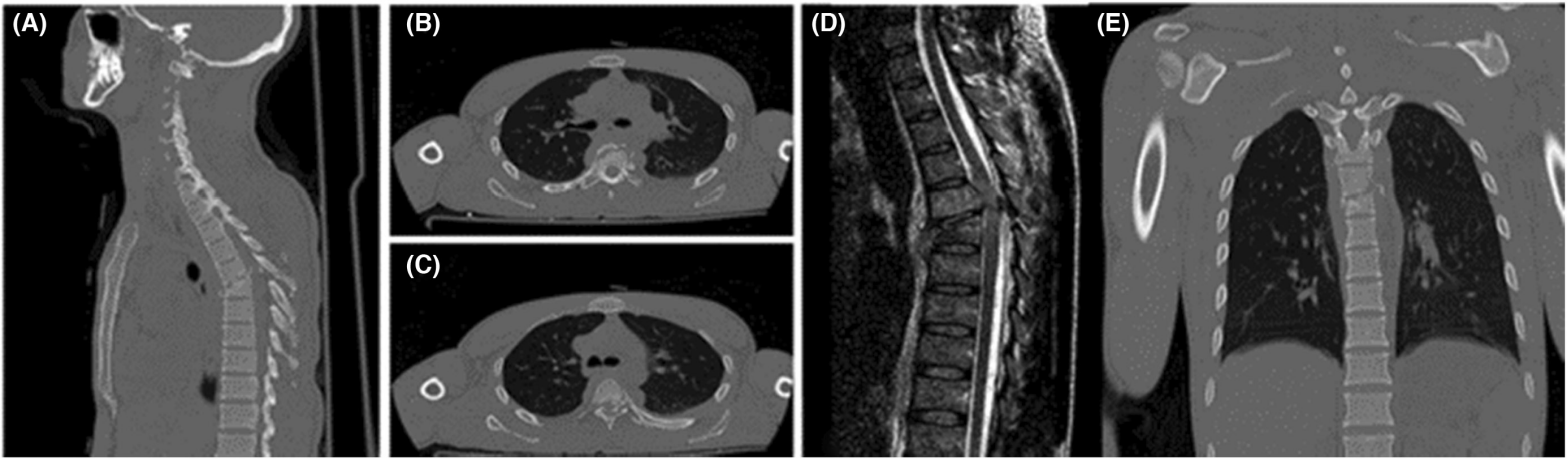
Preoperative computerized tomography (CT) scan and MRI of the thoracic spine show T5 & T6 fractures with kyphotic deformity. (A,B,C,E) Sagittal and Axial CT reconstruction (bone window) of the thoracic spine. (D) MRI sagittal view of the thoracic T2‐weighted sequence shows prominent cord compression
